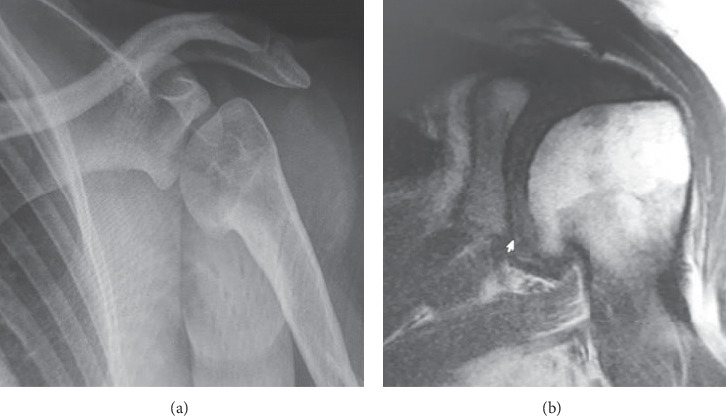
Radiography (a) and magnetic resonance images (b) showing the loss of sphericity associated with osteophytes in the inferior and internal area of the humeral head.
